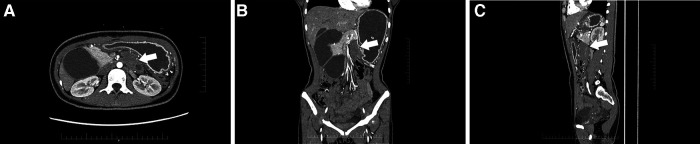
CT revealed a soft-tissue density mass about 5.4 cm*3.2 cm*5.8 cm (arrow), showing inhomogeneous density with ill-defined margin, and having a non-enhancing necrotic area with poor demarcation from the surrounding intestine (A) axial view, (B) coronal view, (C) sagittal view.
